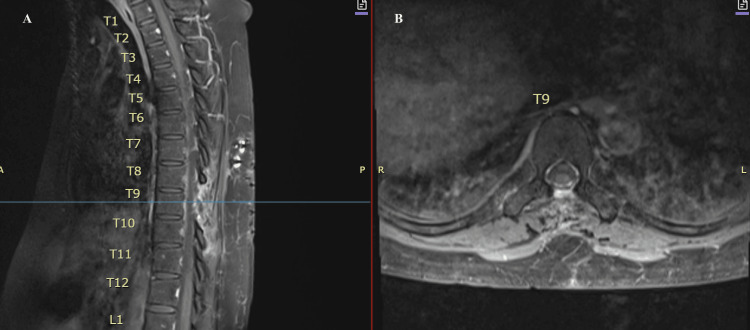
Nine-month postoperative T1-weighted contrast-enhanced MRI of the thoracic spine Sagittal (A) and axial (B) T1-weighted contrast-enhanced MRI images showing resolution of the previously observed small focus of dorsal spinal cord edema at T9. Mild spinal cord volume loss is present at the T9 level. Enhancement along the dorsal thecal sac at T9 is decreased, indicating probable postoperative improvement.
